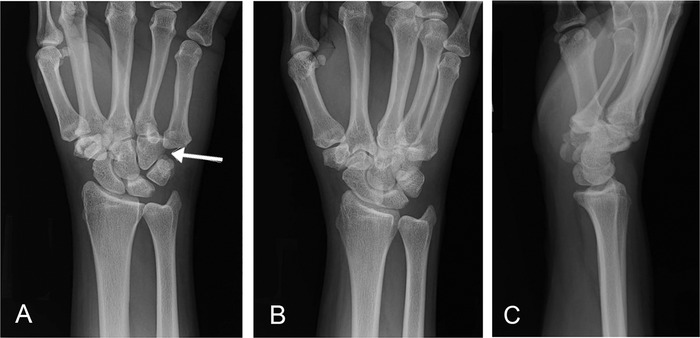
Follow-up wrist posteroanterior (A), oblique (B), and lateral (C) x-rays in the emergency department confirmed an isolated volar and ulnar dislocation of the fifth metacarpal base.
